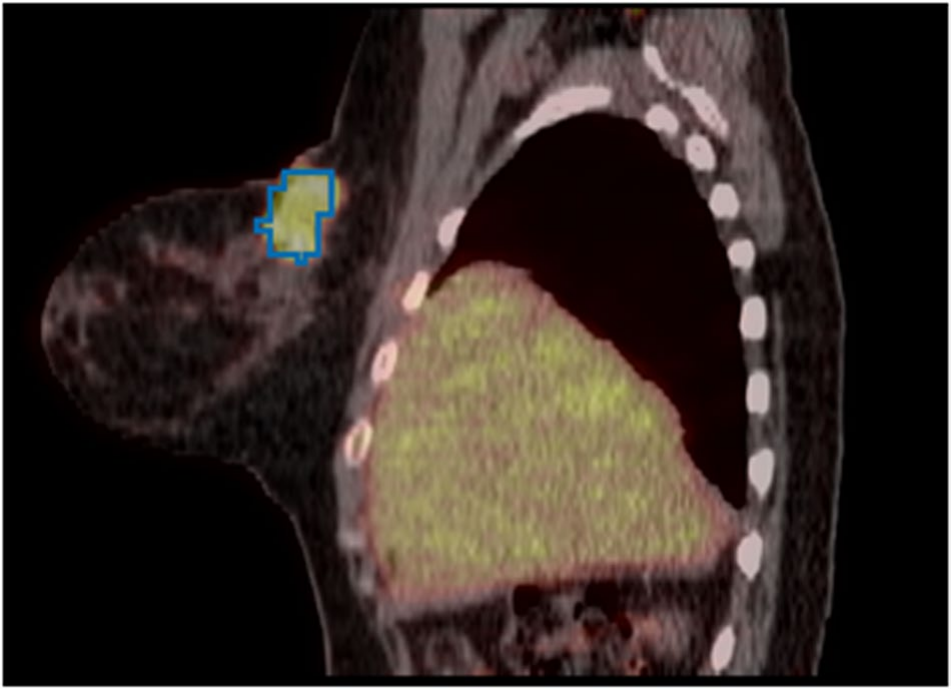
Automatic segmentation of FDG-avid breast tumor (blue) from FDG-PET/CT scans of patient QIN-BREAST-01-0033.
